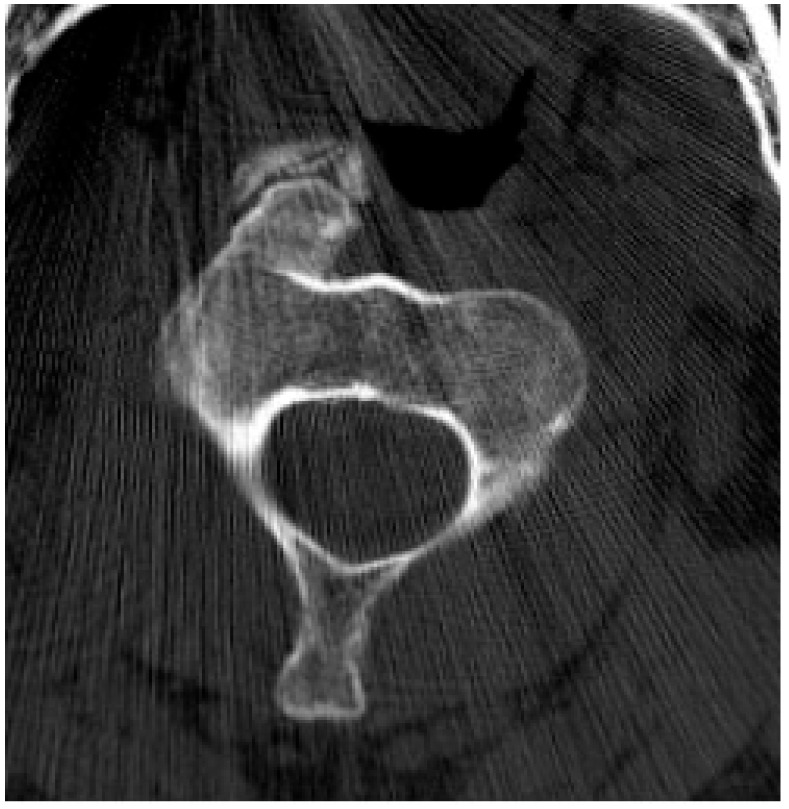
Osteochondroma. Axial CT bone window, lesion extending from the anterior aspect of the vertebral body with the medullary cavity and cortex congruent with the normal adjacent bone.
